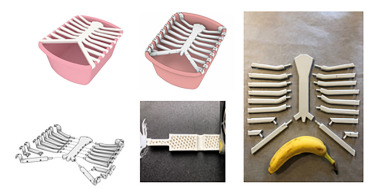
Clockwise: Blind approach pericardiocentesis anterior chest model; ultrasound-guided pericardiocentesis rendering; de-atomized model with scale; cross-section of printing lattice; and de-atomized rendering.
